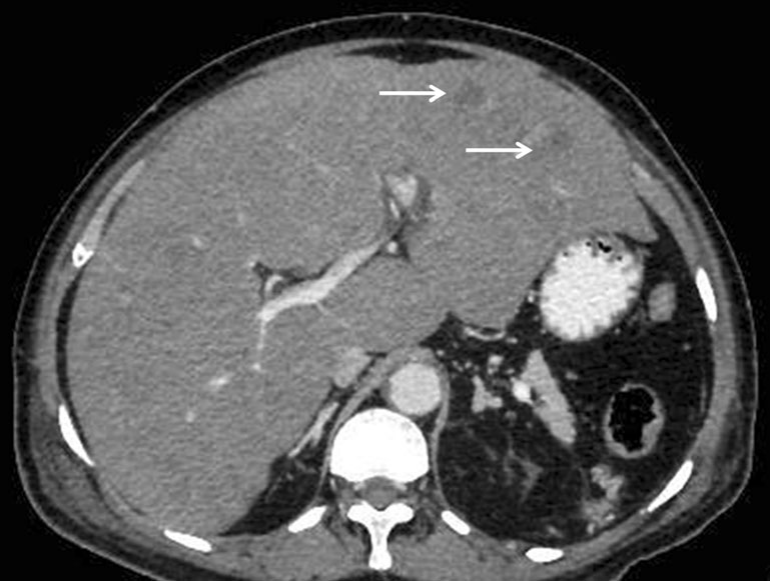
Mixed infiltrating and nodular variety of SHL in a 57-year-old man. Axial CECT image showing ill-defined hypoenhancing lesions in the left lobe of liver (arrows) on a background of hepatomegaly. Liver biopsy revealed lymphomatous infiltration of hepatic sinusoidsPeriportal massPeriportal spread of lymphoma can be explained by the fact that lymphatic vessels along the portal vein and bile ducts are responsible for 80 % of hepatic lymph drainage [45]. This variant is being increasingly recognized and manifests in the form of periportal soft-tissue cuffing or ill-defined mass [46–48]. Both primary and secondary forms of hepatic lymphoma can present in this manner.When the involvement is limited to periportal soft tissue cuffing, this variety of hepatic lymphoma should be differentiated from the other causes of “periportal halo” like biliary dilatation, lymphedema (secondary to transplantation or portal lymphadenopathy), trauma, cardiac failure, and AVH. Out of these, AVH can be particularly difficult to differentiate from lymphoma on CT and MRI because in both these conditions, patients present with hepatosplenomegaly and periportal cuffing in the setting of deranged liver functions. However, US is helpful in such cases because periportal cuffing in lymphoma is usually hypoechoic while in AVH, it is echogenic (Fig. 14). Additionally, in AVH, direct and indirect bilirubin levels are usually elevated in roughly equal proportions and ALP and lactate dehydrogenase (LDH) levels are normal or only mildly elevated. In contrast, moderate elevation of ALP and LDH can occur in lymphoma due to tumour infiltration or extrahepatic bile duct obstruction with resultant direct hyperbilirubinemia. Also, in lymphoma, only a mild increase in serum transaminases is seen while AVH causes significant elevation in serum transaminase levels, often reaching up to 100 times their normal ranges. Viral markers can also used to differentiate between the two, although the coexistence of Hepatitis-B and C infection in patients with lymphoma limits its diagnostic utility. Bile duct dilatation can be differentiated from lymphoma by the complete encasement of the portal vessels by the tumour and its hypoechoic appearance as opposed to the anechoic biliary radicals. Other causes of periportal halo can usually be diagnosed on the basis of clinical history.
